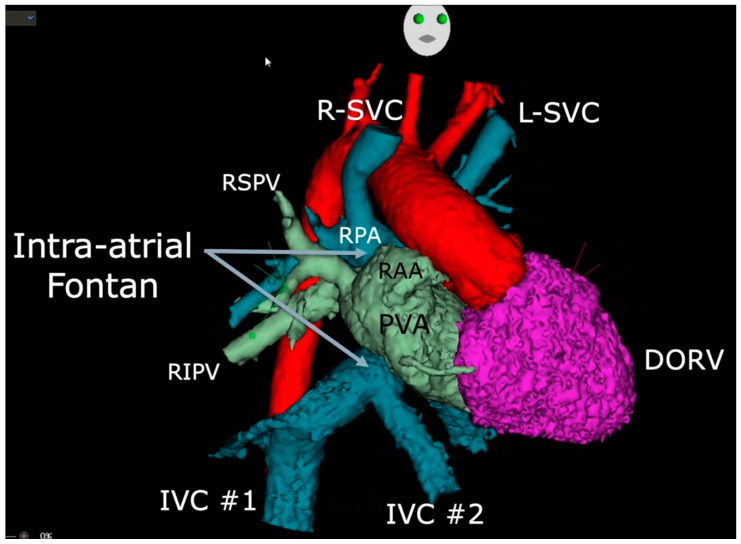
CT scan images segmented and imported into a 3D-electroanatomic mapping system. R-SVC and L-SVC denote right and left superior vena cavae; RSPV, right superior pulmonary vein; RPA, right pulmonary artery; RAA, right atrial appendage; RIPV, right inferior pulmonary vein; PVA, pulmonary venous atrium; DORV, double-outlet right ventricle; IVC, inferior vena cava.
